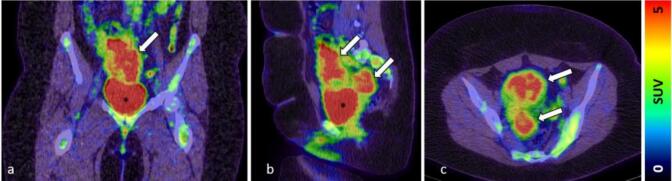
PET/CT (206 MBq ^18^F-FDG) a coronal fusion, b sagittal fusion, c transaxial fusion; *:bladder; white arrows: Omental tumor lesions with increased glucose metabolism compatible with malignancy (SUV: standardized uptake value).
